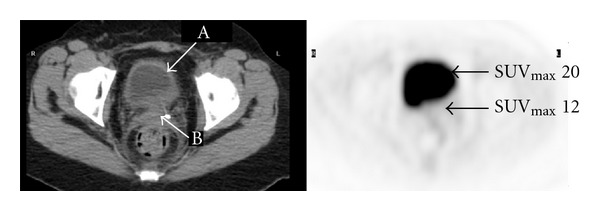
Transaxial FDG PET-CT images of the pelvis show no abnormal uptake in the endocervix of a woman status after chemoradiation for cervical cancer. However, measured SUV_max⁡_ is 12 in the endocervix (arrow B). Urine SUV_max⁡_ in the bladder is 20 (arrow A).
